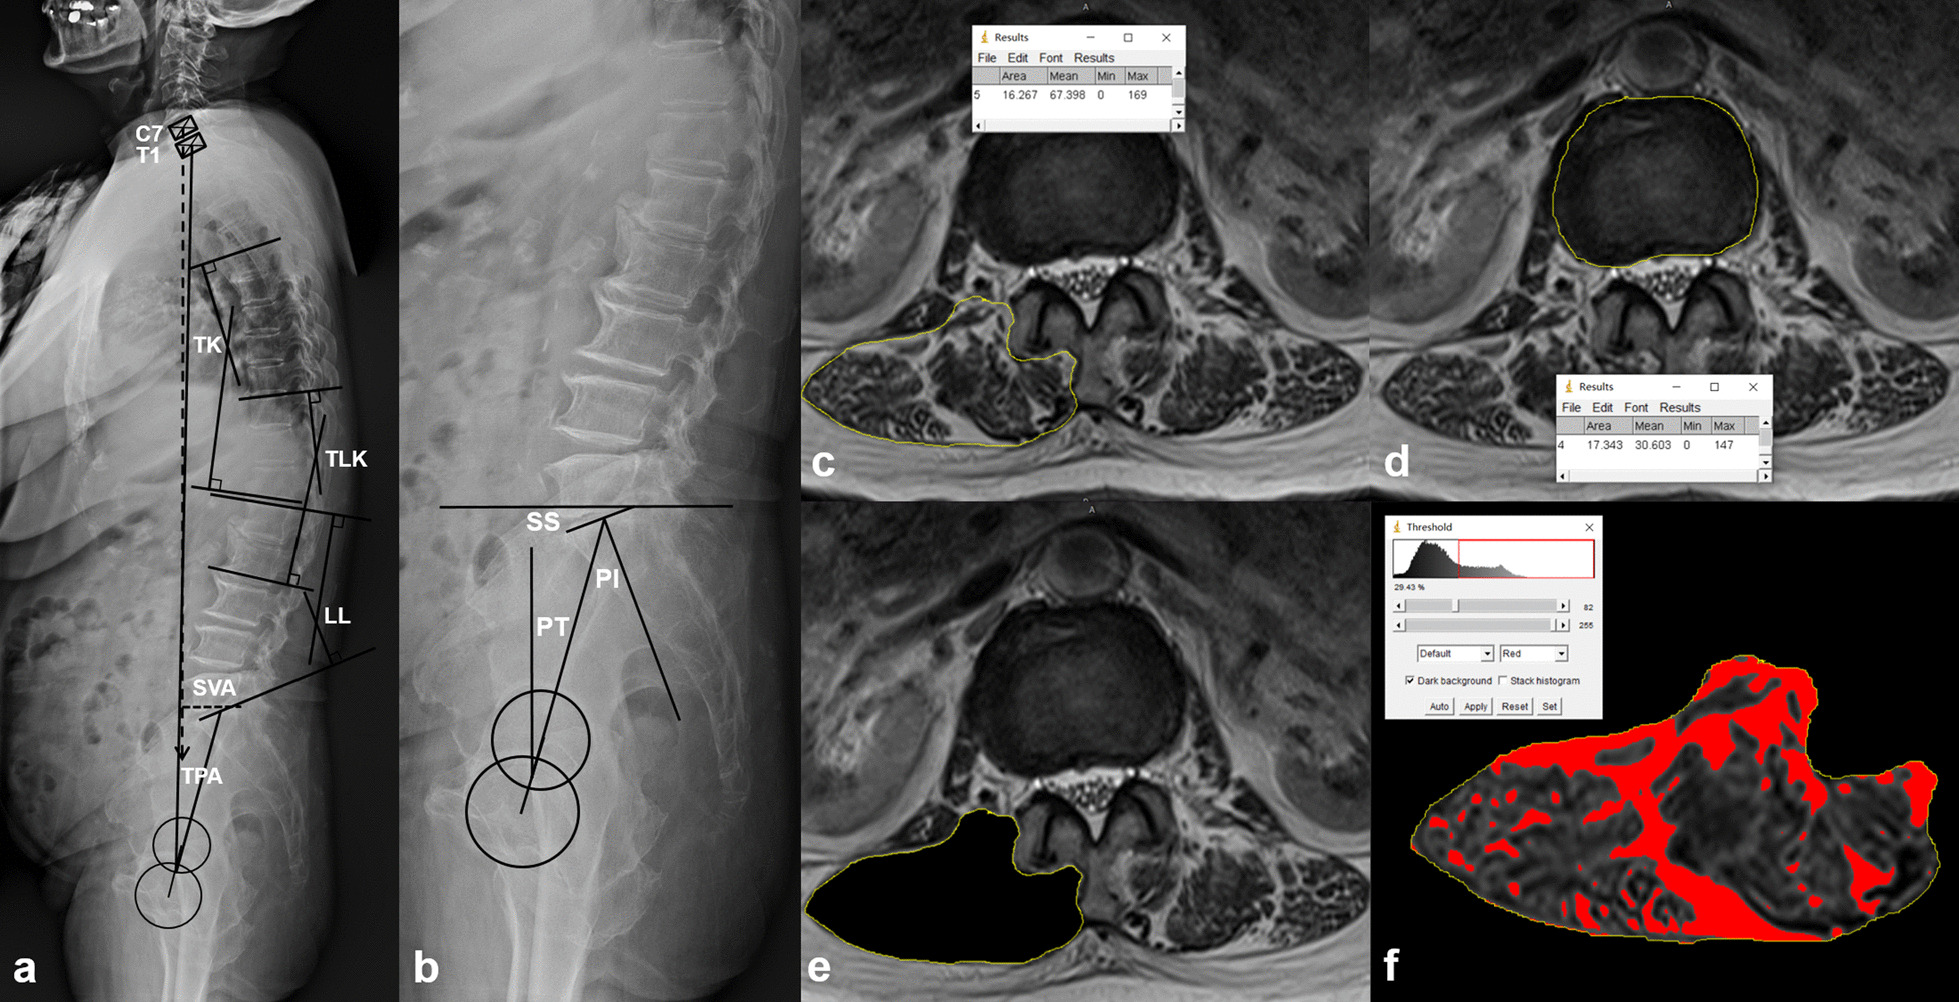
Spinopelvic parameters measurement and back muscle evaluation. a Measurement of spinal parameters. b Measurement of pelvic parameters. c The fascial boundary of lumbar paravertebral muscles (yellow circle): the fascia thoracolumbalis was traced down laterally and anteriorly to the dorsal side of the quadratus lumborum, followed by the posterior surface of the facet and lamina, and lateral margin of spinous process. d The boundary of vertebral body (yellow circle). Muscle/disk ratio: 16.267/17.343 = 0.94. e Cut the muscle along the fascial boundary. f Bright pixels of fat tissue in the MR images were colored in red (darker color in the black and white version) using pseudocoloring technique. The percentage of the red pixel area in the muscle compartment was the percentage of fat infiltration (29.43%)
